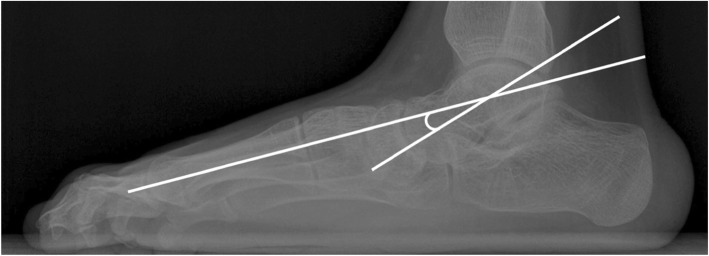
The lateral talo-first metatarsal angle is the angle between the line bisecting the longitudinal axis of the first metatarsal bone and the line bisecting the longitudinal axis of the talus on the standing lateral plain foot radiograph
